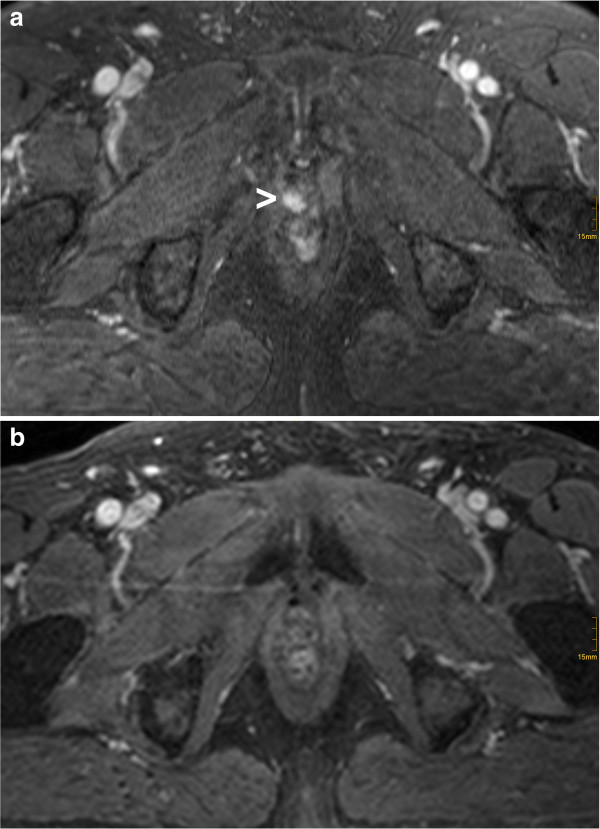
Example of a patient classified as true positive. A contrast enhancing lesion was detected on pre-RT DCE-MRI (a) (arrowhead), with completely morphologic response on the post-RT DCE-MRI (b).
